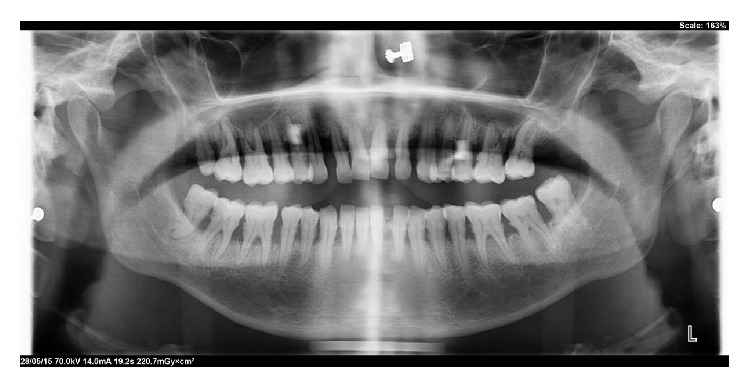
Orthopantomogram showing normal bone morphology.
